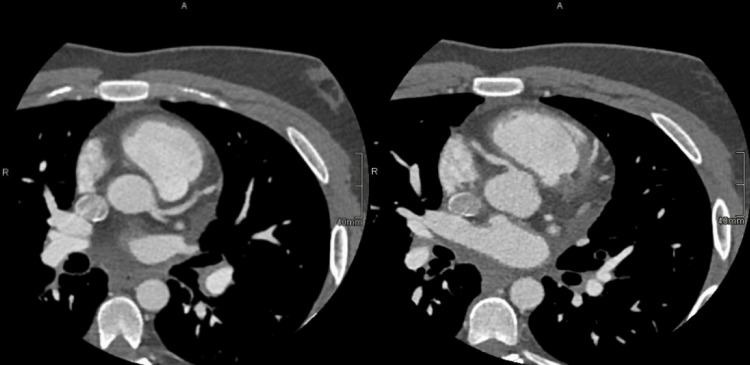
Axial view of the coronary CT angiogram (Coro-scan) of the younger sibling showed normal and patent coronary arteries, favoring the diagnosis of myocarditis.
